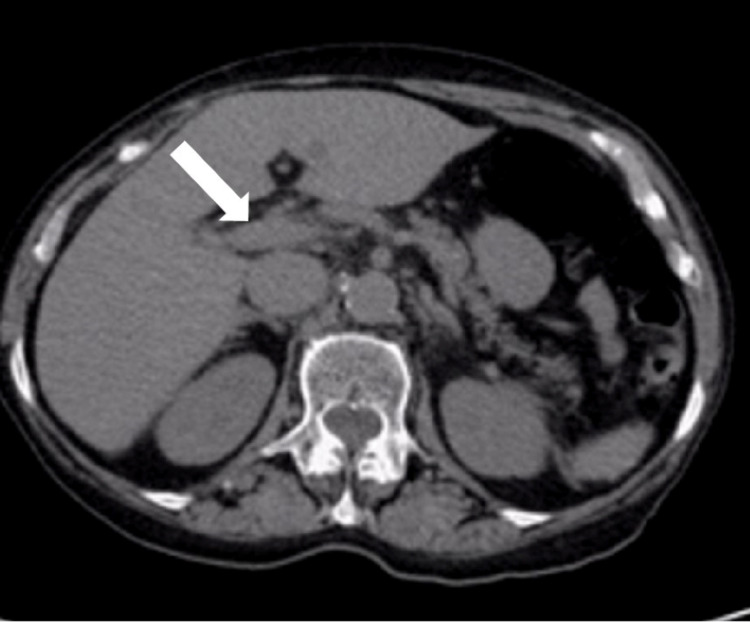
Abdominopelvic CT performed in the emergency department, revealing enlarged hepatic veins (white arrow) and signs of congestive hepatopathy. The exam also excluded the presence of biliary tract obstruction.
